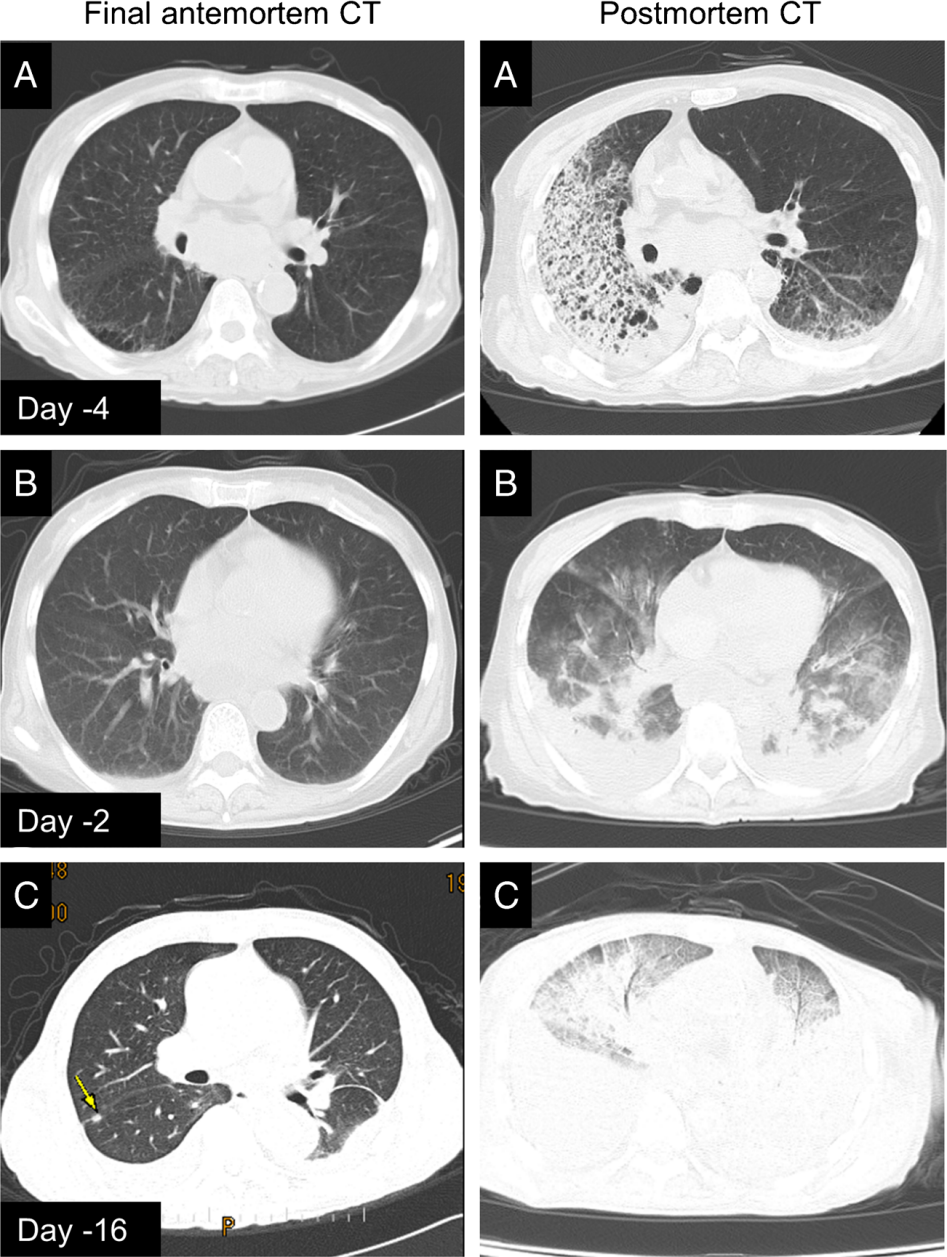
Three representative sets of final antemortem CT and postmortem CT images having dramatic changes in the lung fields. Case *A* represents bronchopneumonia, predominantly infiltrated in the right lung fields for 4 days. Case *B* shows acute respiratory distress syndrome (ARDS) with bilateral pleural effusion documented for only 2 days at the agonal phase in a patient with acute leukemia. Case *C* shows a patient with a solid malignancy who was considered by the attending physicians to have died due to cancer progression while the immediate cause of death was diagnosed as ARDS by postmortem CT. Yellow arrow: metastatic tumor. *CT* computed tomography
